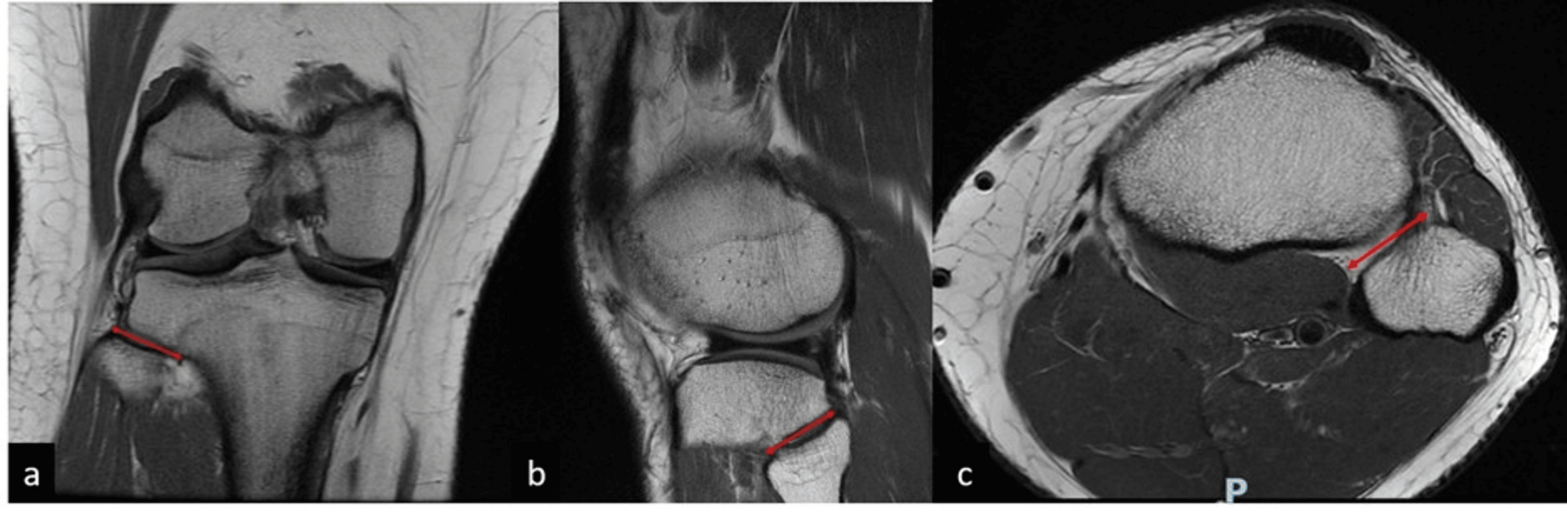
MRI imaging of the right knee in the coronal (a), saggital (b), and axial (c) views. The proximal tibiofibular joint width, demarcated in arrowed red lines is measured.
